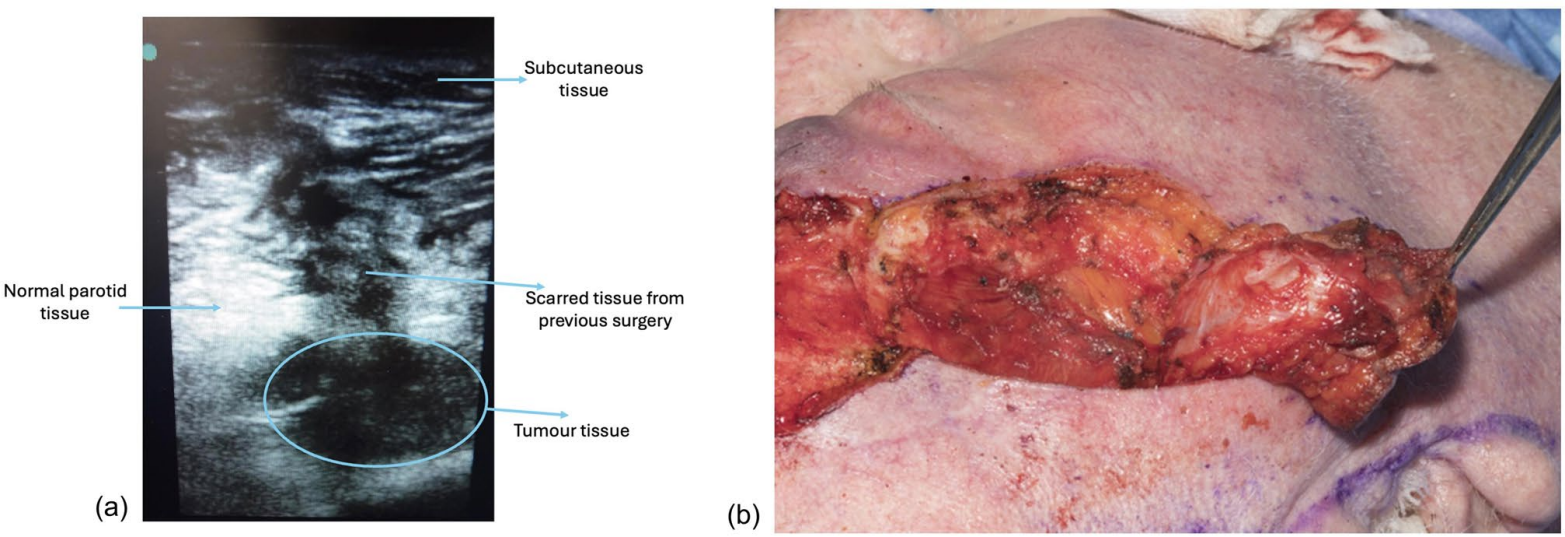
Intraoperative images including (a) ultrasound scan and (b) tumour resection and residual defect
